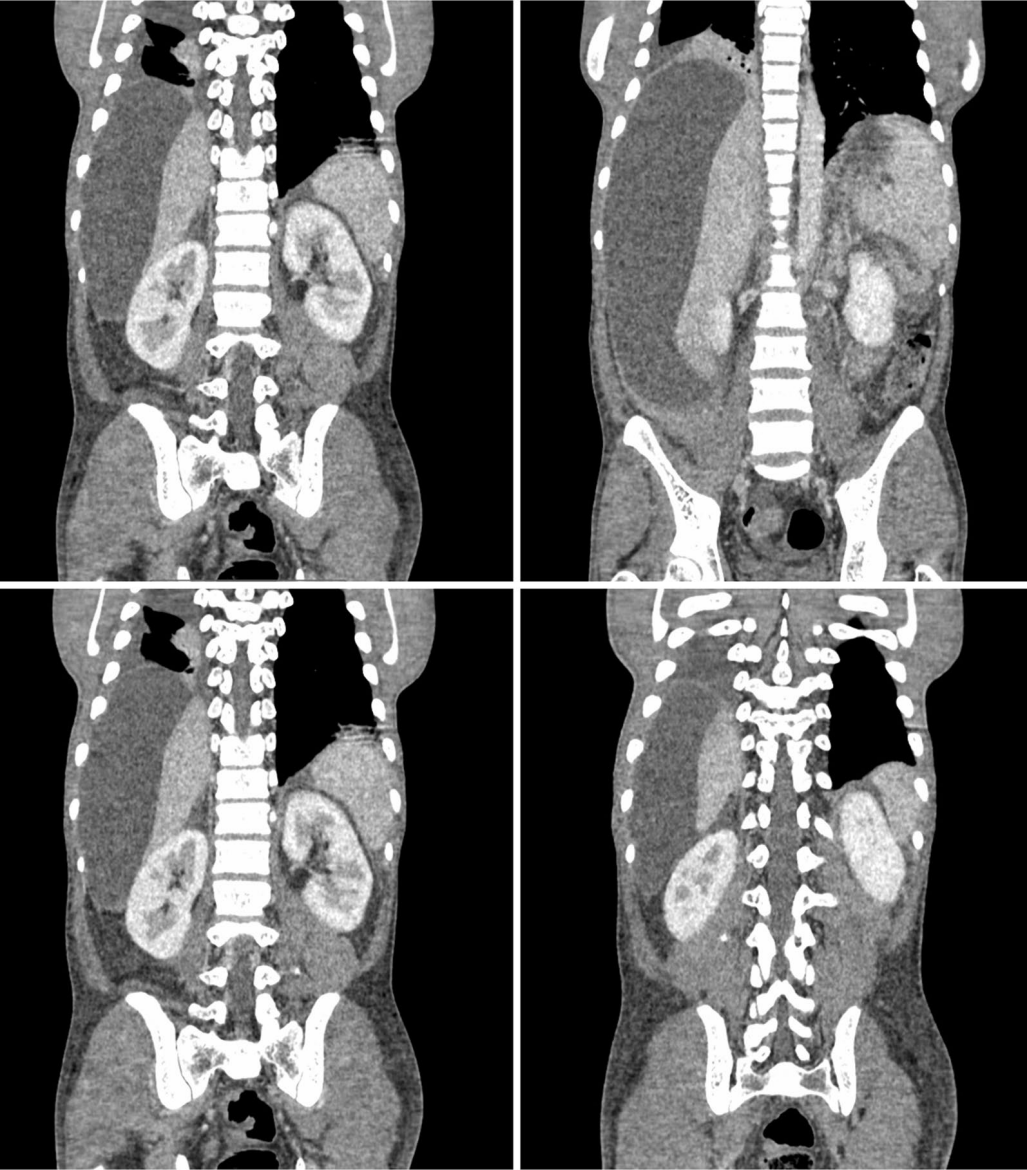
Contrast enhanced CT scan of the abdomen—coronal view
